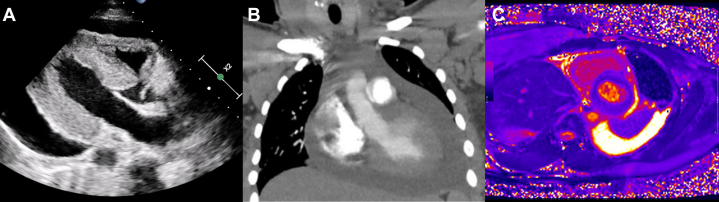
Cardiac Tamponade as Index Presentation of SLE in Postpartum Period A 35-year-old patient presented to the accident and emergency department with symptoms of breathlessness in the postpartum period and was found to be have cardiac tamponade on transthoracic echocardiography (A), and with a large pericardial effusion on computed tomography (B). Later, after pericardiocentesis, the patient underwent a cardiac magnetic resonance that demonstrated myocarditis (C) and was diagnosed with systemic lupus erythematosus. Anti-inflammatory therapy was initiated and the patient made a full recovery.
